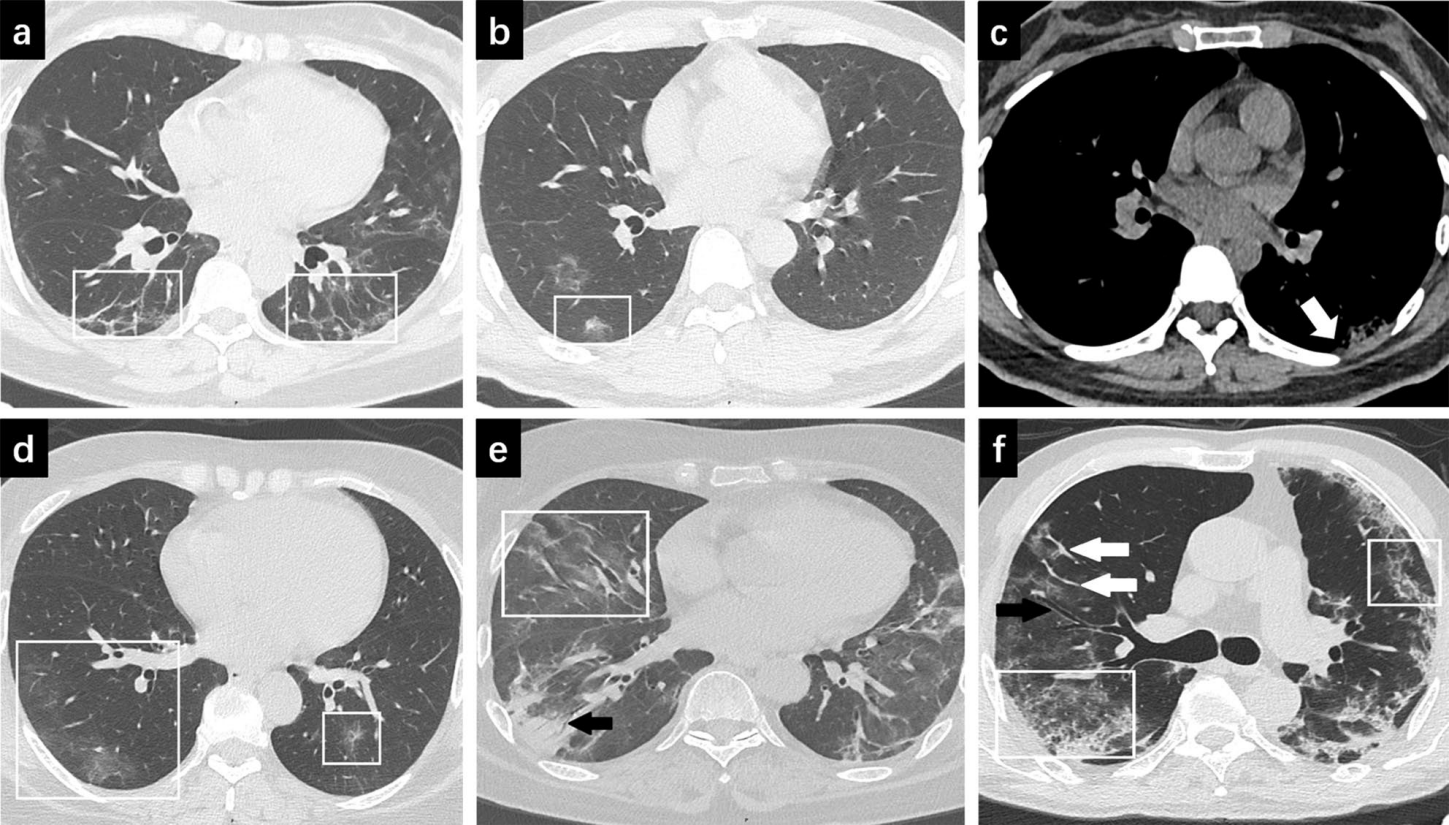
CT images of patients with COVID-19. (a) 56-year-old woman with moderate COVID-19. CT image shows pulmonary fibrosis in both lungs (box). (b) 37-year-old man with moderate COVID-19. CT image shows mixed ground glass opacity (box). (c) 32-year-old woman with moderate COVID-19. CT image shows pleural thickening with pleural adhesion (arrow). (d) 50-year-old woman with severe COVID-19. CT image shows ground glass opacities in both lungs (box). (e) 59-year-old woman with severe COVID-19. CT image shows ground glass opacities (box) and consolidation with air bronchogram (arrow) in the right lung. (f) 65-year-old man with severe COVID-19. CT image shows bronchial wall thickening and bronchiectasis (black arrow). Vascular enlargement is also shown (white arrows). The two boxes show pulmonary interstitium reticular thickening in both lungs.
